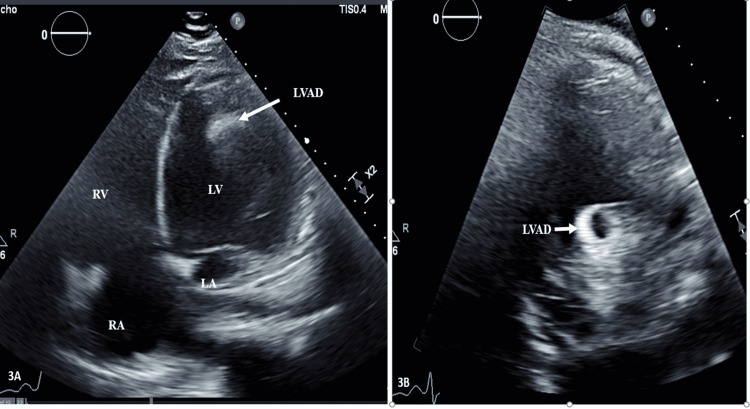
Four-chamber apical view and parasternal long view demonstrating severely dilated left ventricle function with LVAD in place extending from the left ventricle (LV), dilated left atrium (LA), right ventricular (RV) dysfunction, and the right atrium (RA).
